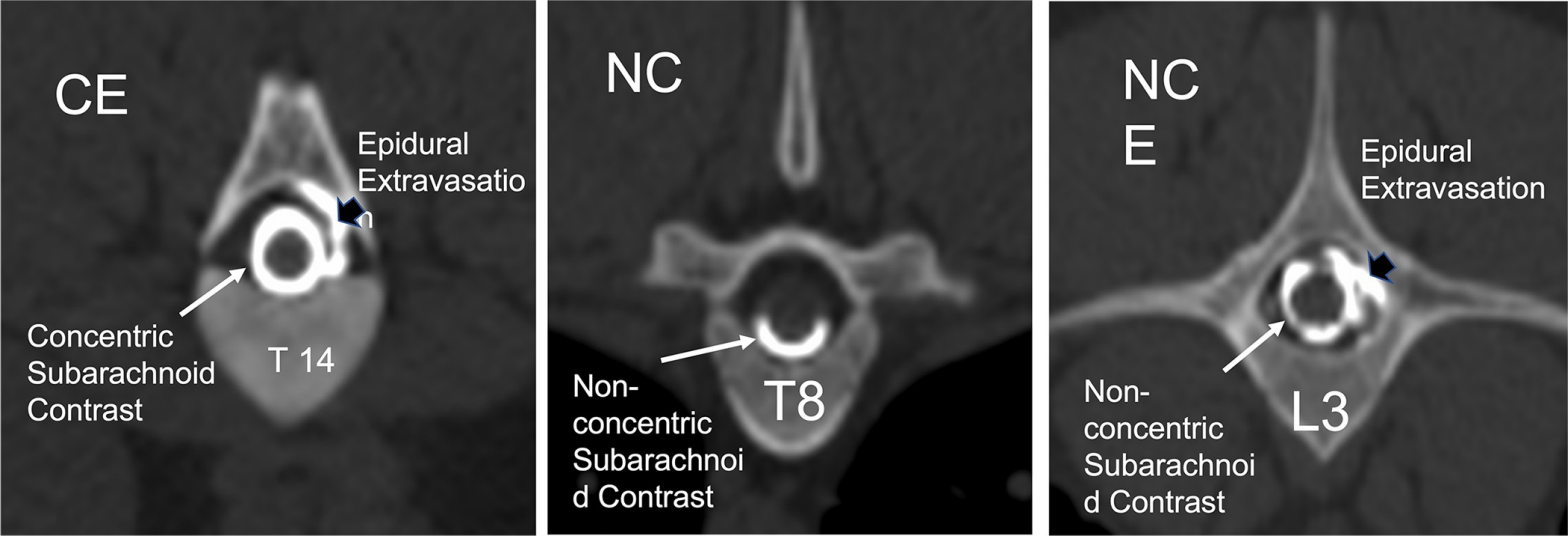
Transverse CT myelogram images showing the qualitative descriptions for contrast filling morphology. (C = concentric, NC = Non-concentric) and presence of epidural extravasation (E = epidural extravasation), or a combination of epidural contrast and non-concentric filling (NCE) or CE for concentric with epidural extravasation.
